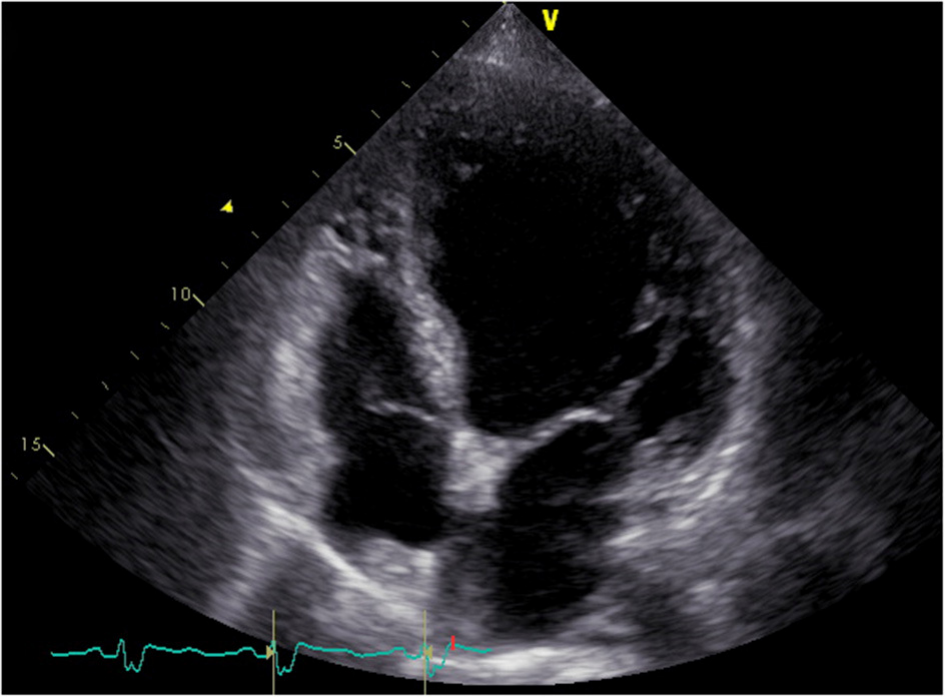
Four-chamber echocardiogram showing a dilated left ventricle.
